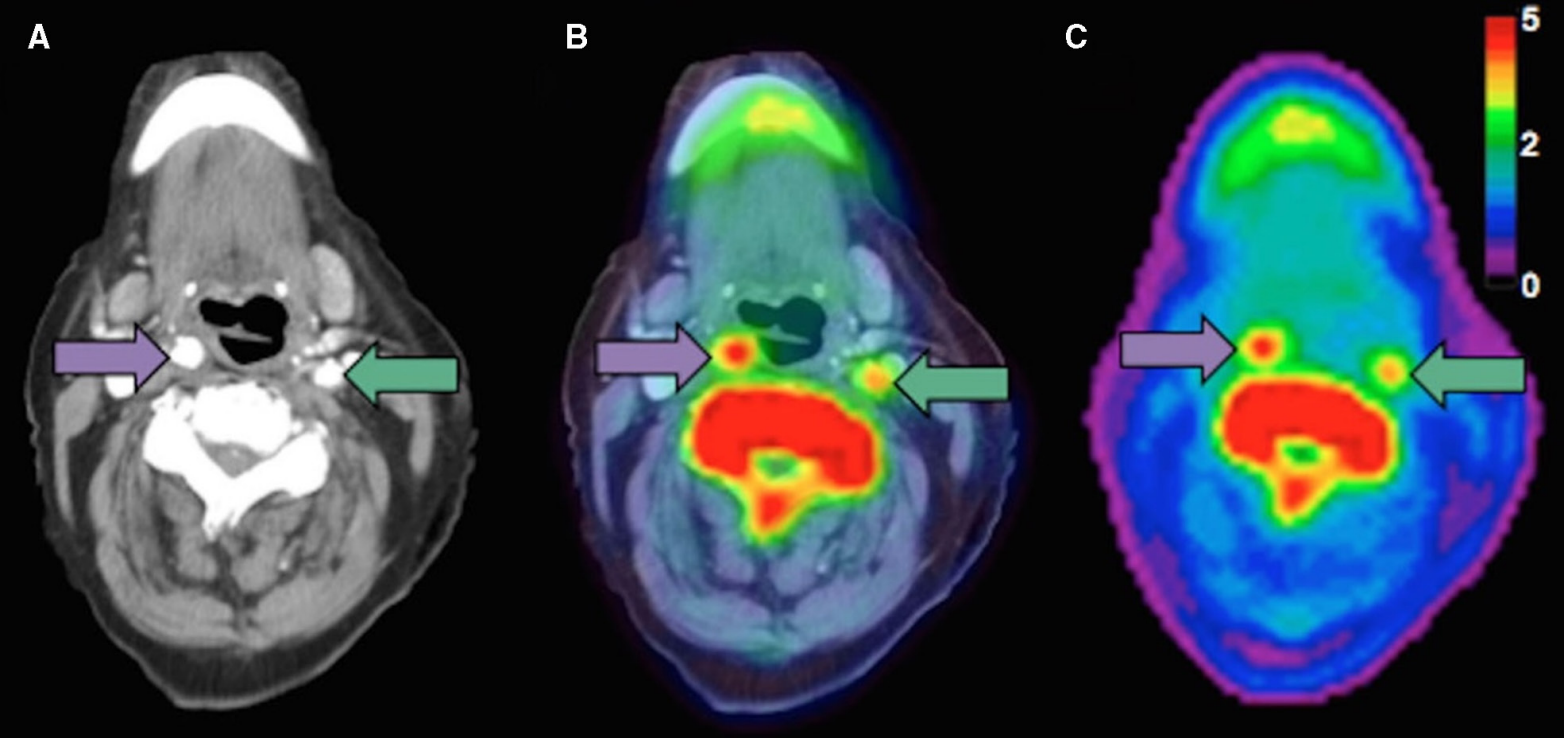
Tracer uptake in symptomatic disease. A, Axial computed tomography angiography (CTA). B, Axial positron emission tomography (PET)/CTA. C, Axial PET showing a symptomatic right carotid artery (purple arrow) and an asymptomatic left carotid artery (green arrow). Adapted with permission from Evans et al.^14^ Copyright © 2020 The Authors. This is an open access article under the terms of the Creative Commons License CC BY 4.0 (https://creativecommons.org/licenses/by/4.0), which permits use and distribution in any medium, provided the original work is properly cited.
